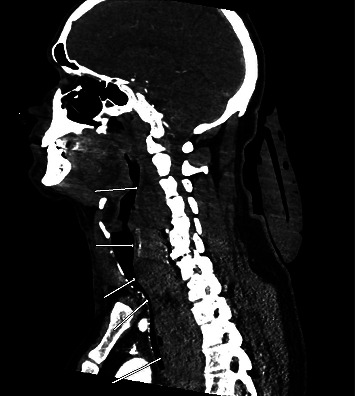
CT image demonstrating a large prevertebral soft tissue hematoma extending from C3 through the carina.
